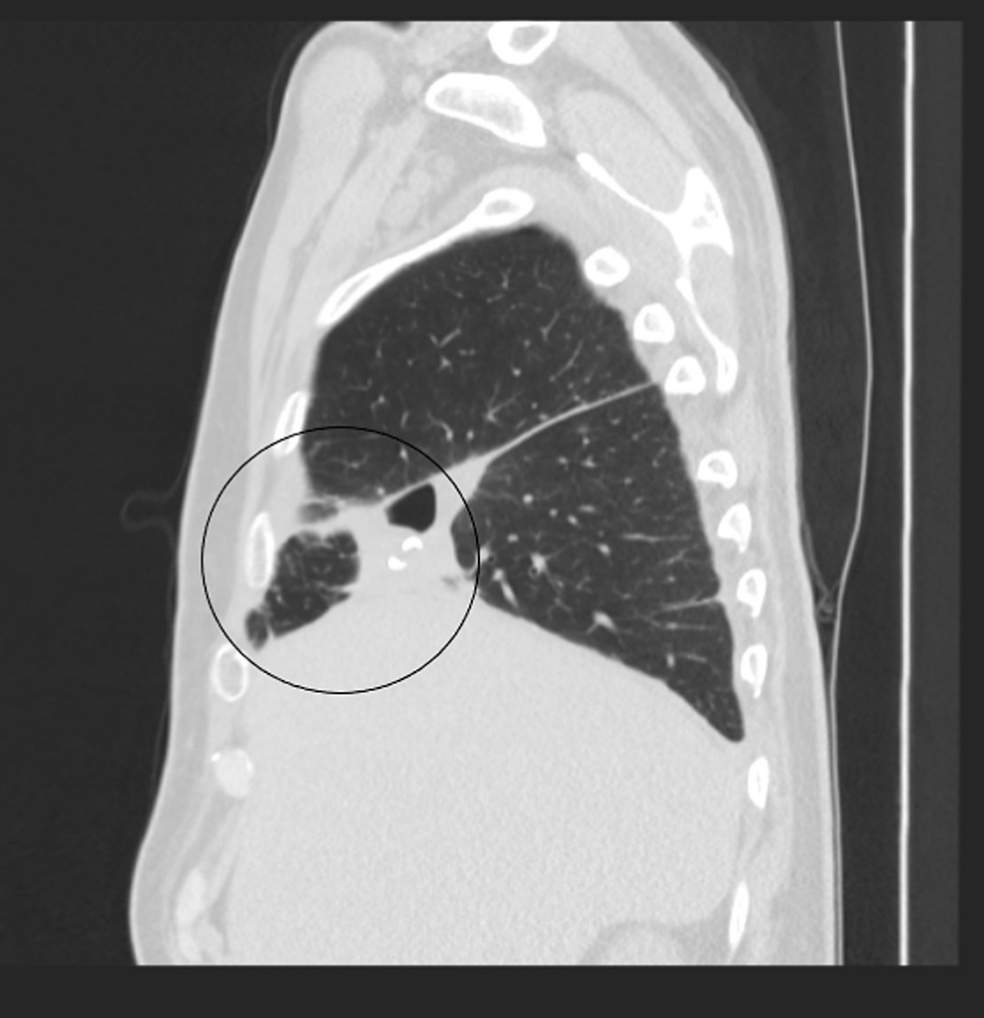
CT of the chest one month later shows a small residual collection of air and possible fluid in the right lateral lower lung near the major fissure in the region of the previous large abscess.
